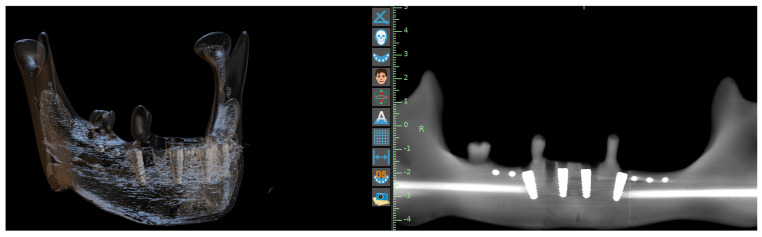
CBCT after implantation, markers and implants visible.
